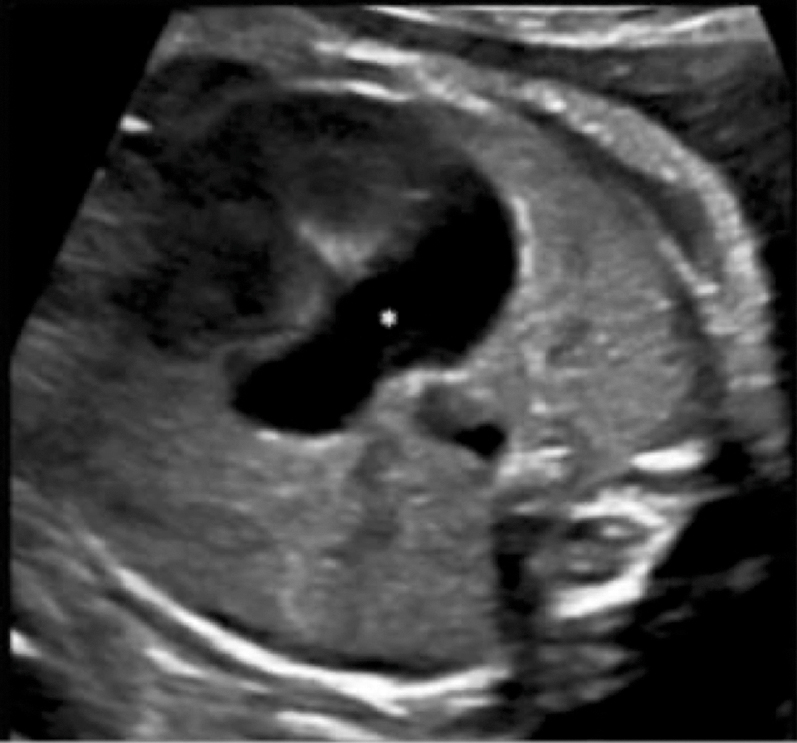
Fetal Echocardiography Demonstrating Enlarged Structure Along the Left Posterior Atrioventricular Groove Measuring 1.5 × 2.2 cm (Asterisk) Inferior sweeps localize the structure to the region of the coronary sinus.
